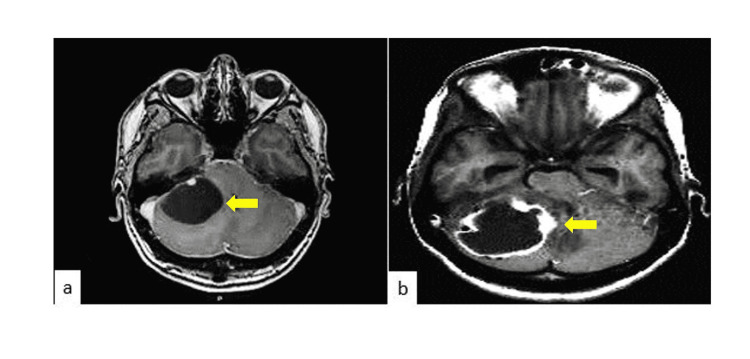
Axial view of the MRI of the brain a: T1 weighted image (T1W), b: T2 weighted image (T2W) T1W, T2W and FLAIR images show a well-defined cystic lesion at the left cerebellum hemisphere of the brain. The T1W axial and T2W coronal images show a well-defined cystic lesion from the dorsally located mural nodule.
